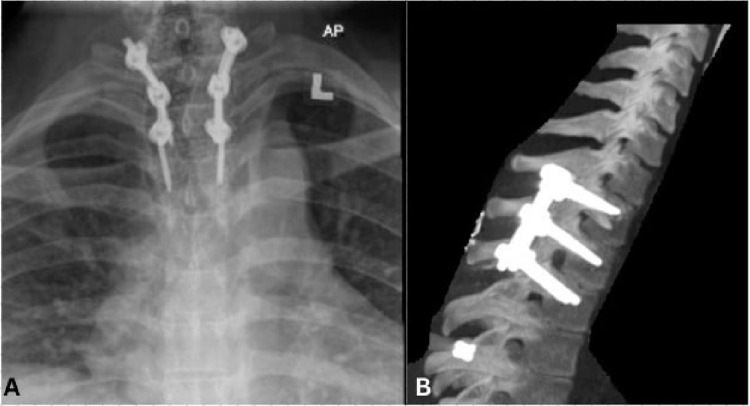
Post-operative AP (A) and lateral (B) radiograph shows a good alignment of the spinal column following posterior pedicle screw fixation.
